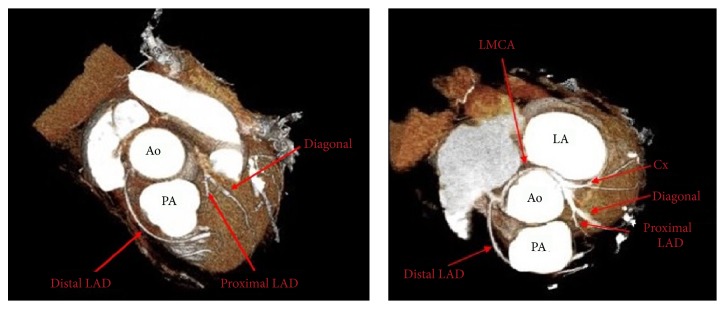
Colored three-dimensional (3D) volume rendered CT angiography images showing single coronary artery (SCA) arising as a common trunk from the right sinus of Valsalva and bifurcating into the right coronary artery (RCA) and the left main coronary artery (LMCA). RCA gives rise also to distal LAD which courses anterior to the PA on its way to the left ventricle. LMCA crosses the base of the heart turning posteriorly behind the aorta dividing into proximal left anterior descending artery (LAD) and circumflex artery. Left atrium (LA), aorta (Ao), and pulmonary artery (PA).
